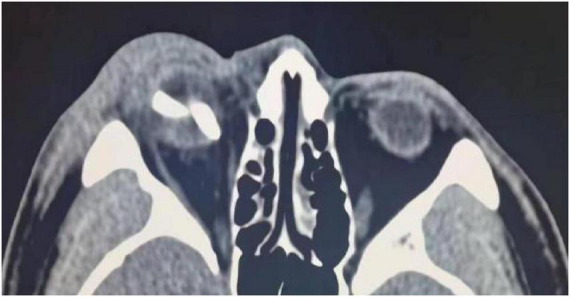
A computed tomography (CT) scan revealed an irregular contour of the right eyeball, indicating the potential presence of intraocular foreign bodies (IOFBs).
